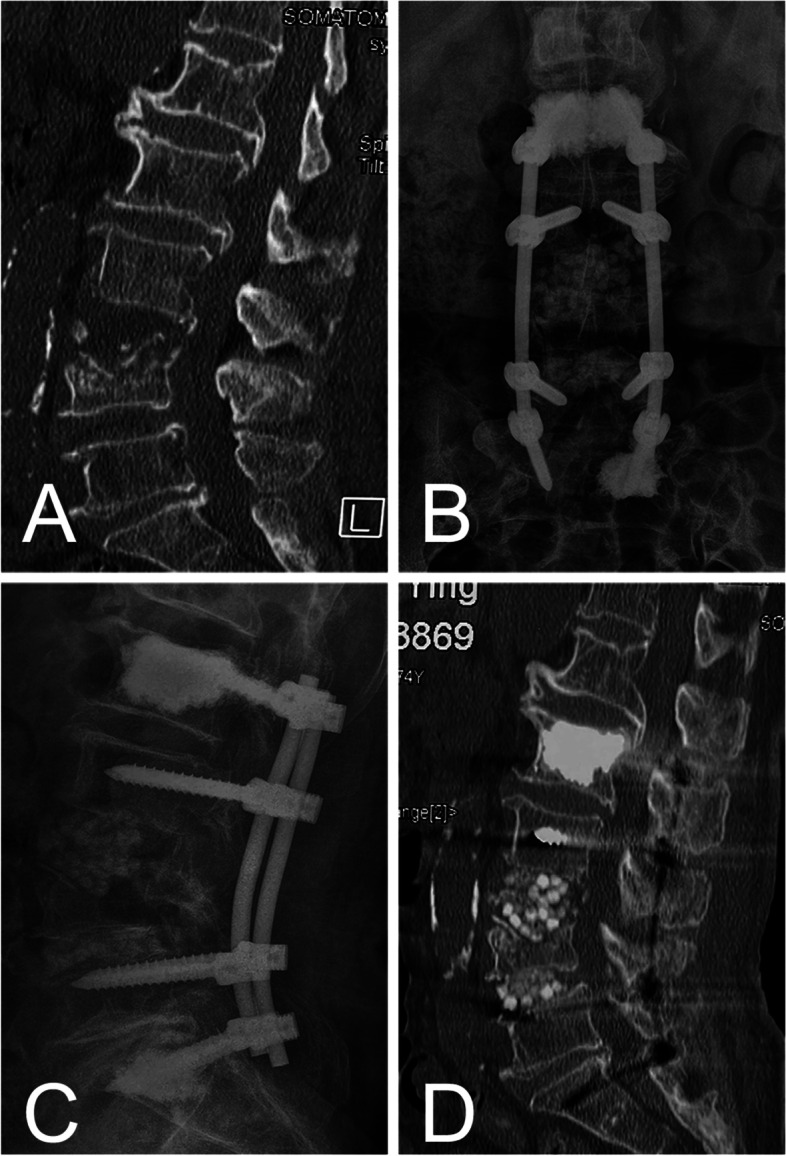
A 74-year-old female suffered from spondylodiscitis with severe osteoporosis. A Preoperative sagittal CT showed severe bone destruction. B,C,D Postoperative anteroposterior and lateral radiographs and sagittal CT showed the bone cement enhanced screw fixation and calcium sulfate beads buttressing the anterior column
